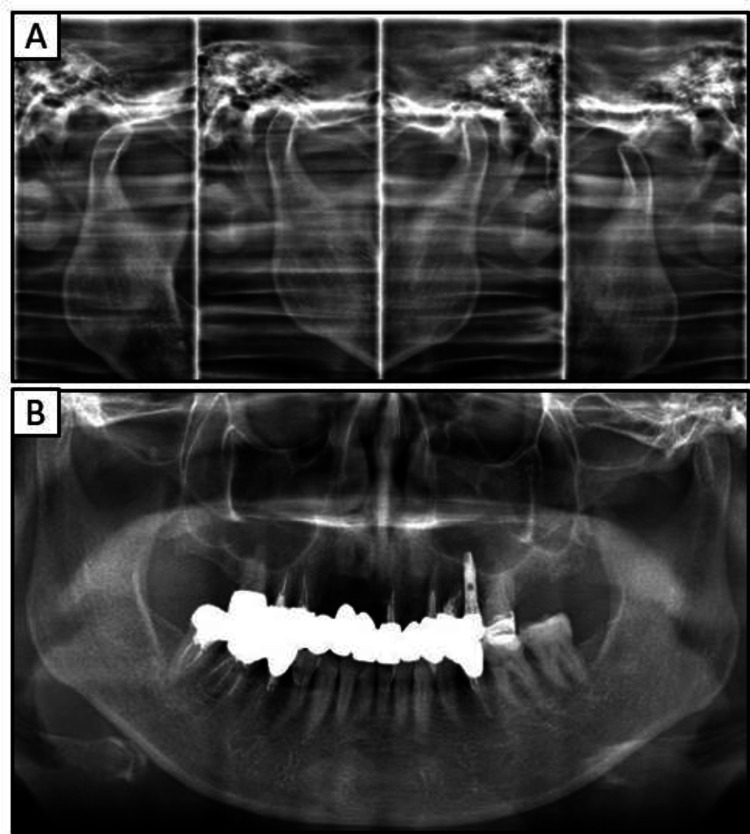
Postoperative radiographs (A) Radiographic image of temporomandibular joint (B) Panoramic radiograph
